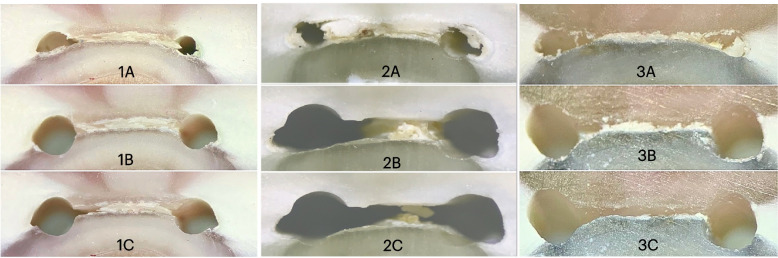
Digital images showing root sections at 2 mm from WL (A) before instrumentation (B) after instrumentation (C) after final irrigation with (1) NA, (2) PUI, and (3) EDDY. Note that (2C) shows irregularities created in the canal due to the ultrasonic tip coming in contact with the dentinal walls during activation (arrow)
